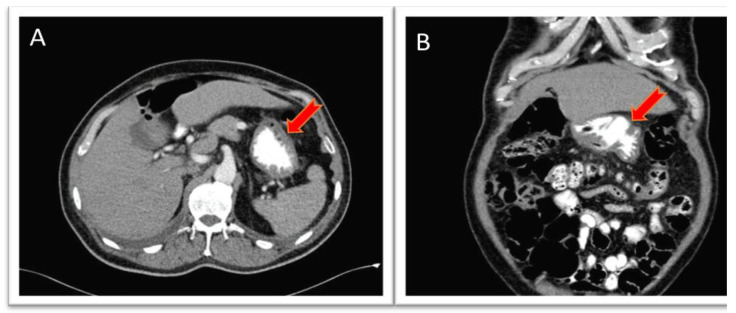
Abdominal computed tomography. (A) The axial view shows a poorly distended gastric chamber; however, a slight thickening of the gastric antrum walls is observed without a defined mass (red arrow). (B) The coronal view also reveals thickening of the gastric antrum walls (red arrow).
